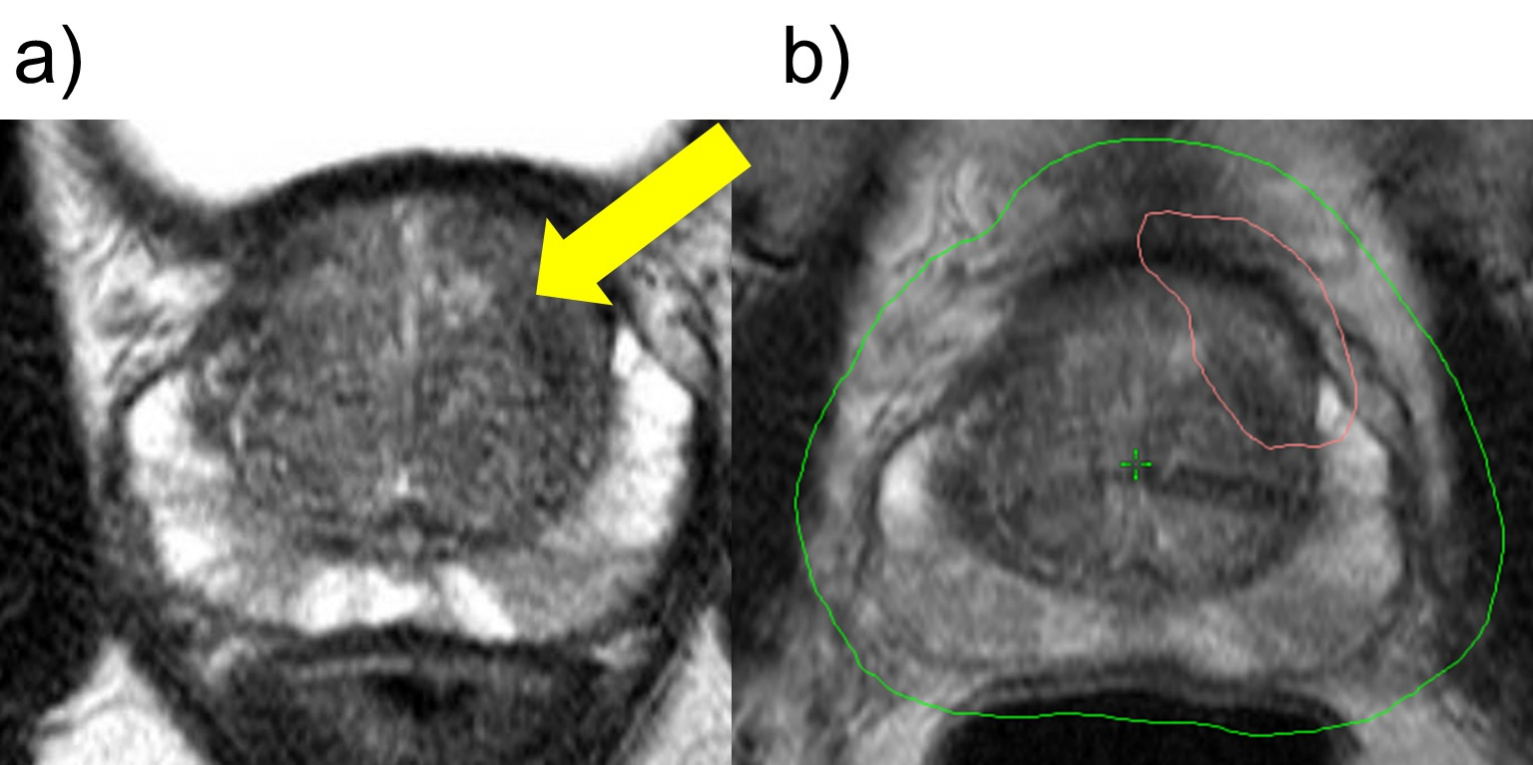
Case 1 shows a left anterior transition zone lesion treated with stereotactic body radiotherapy. (a) Hypointense left anterior transition zone lesion with suspicious PI-RADS 5 score radiographic features (indicated by arrow) on T2 magnetic resonance imaging (MRI). (b) Green and red distributions indicate 36.25 Gy and 40 Gy radiation isodose lines, respectively.
